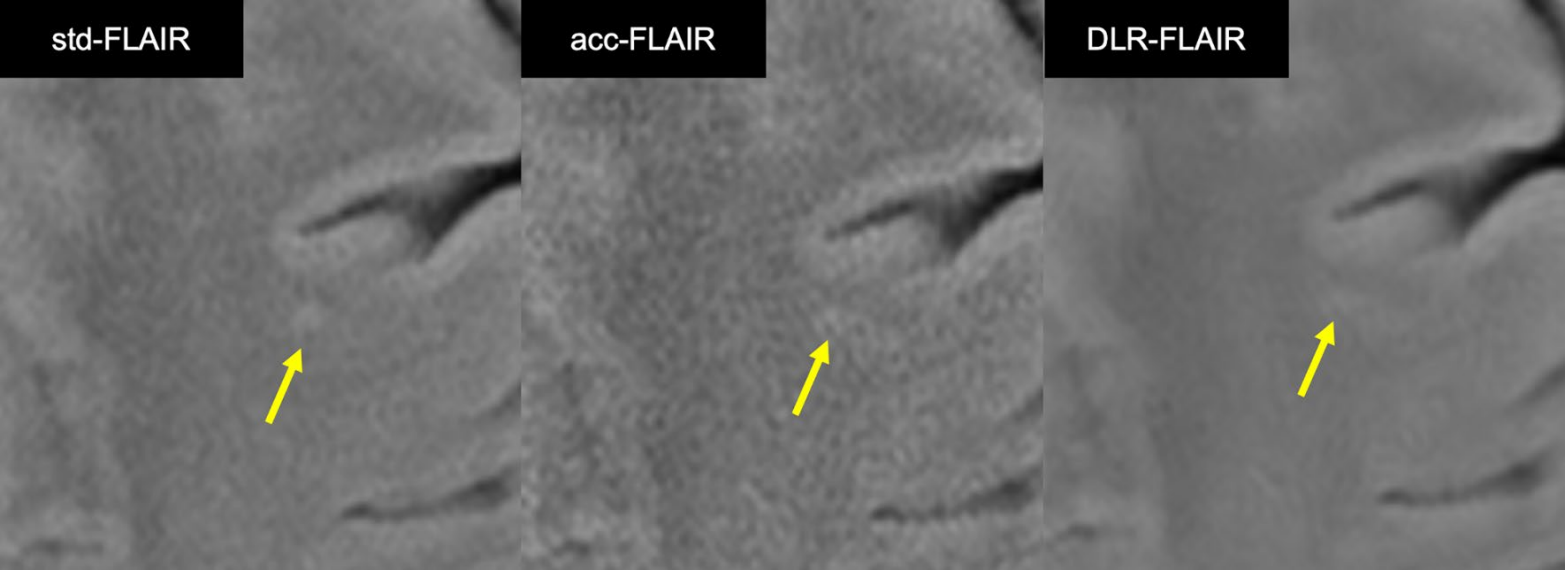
Hyperintensity that appeared differently in acc-FLAIR and DLR–FLAIR compared to std-FLAIR. In the acc-FLAIR image, the hyperintensity shows more noise and appears differently in shape compared to std-FLAIR image. In the DLR–FLAIR image, while the noise is removed, the hyperintensity shape still differs from that in the std-FLAIR image. This hyperintensity was scored 1 by all neuroradiologists for acc-FLAIR and by two neuroradiologists for DLR–FLAIR
